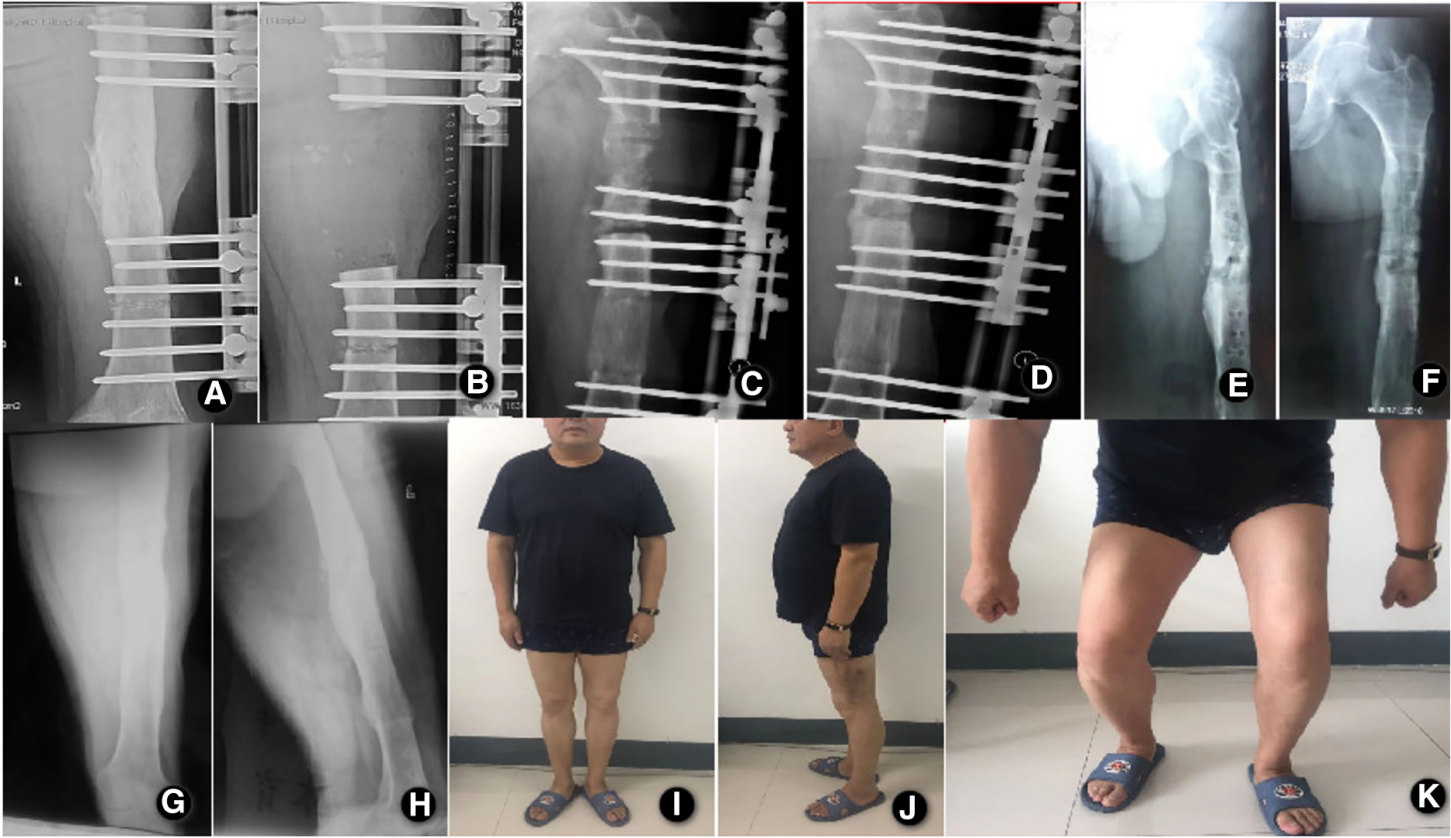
A 42-year-old male patient with a left femur fracture caused by a traffic accident and post-traumatic osteomyelitis after external fixation. (A) Anteroposterior x-ray showed osteomyelitis of the middle femur; (B) Anteroposterior x-ray films on the day after radical debridement and installation of a rail fixator system showed a bone defect in the length of 10.2 cm; (C,D). Anteroposterior and lateral x-ray films 27 days after the operation, showed bone contact was reached; (E,F). Anteroposterior and lateral x-ray films at 13.6 months after the operation, showed good bony union and good regenerate consolidation; (G,H). Anteroposterior and lateral x-ray films show the good bony union and good regenerate consolidation at 12 months after the removal of external fixation. (I,J,K). Functional recovery at 12 months after the removal of external fixation.
